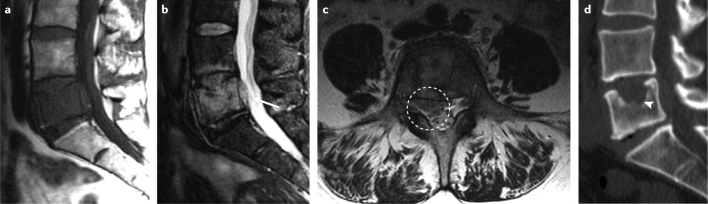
Imaging techniques for RCC bone metastases. Bone metastases of the lumbar spine are detected by MRI or CT. T1-weighted sagittal (part a), T2-weighted sagittal (part b) and T2-weighted axial (part c) MRIs show the destruction of the fifth lumbar vertebra. MRI offers benefits for the assessment of the spinal canal and its possible involvement (arrow and dashed circle). By contrast, CT imaging (part d) has the advantage of displaying the structure of the mineral bone and enables assessment of its stability in the case of osteolysis (arrowhead). RCC, renal cell carcinoma.
